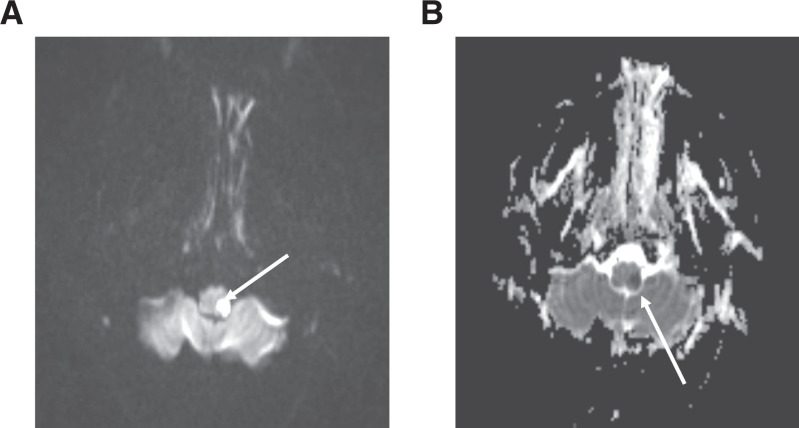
Brain magnetic resonance imaging scan showing infarct in the left lateral medulla with hyperintensity on Diffusion-weighted imaging (A) and hypodensity on apparent diffusion coefficient (B) (white arrow).
